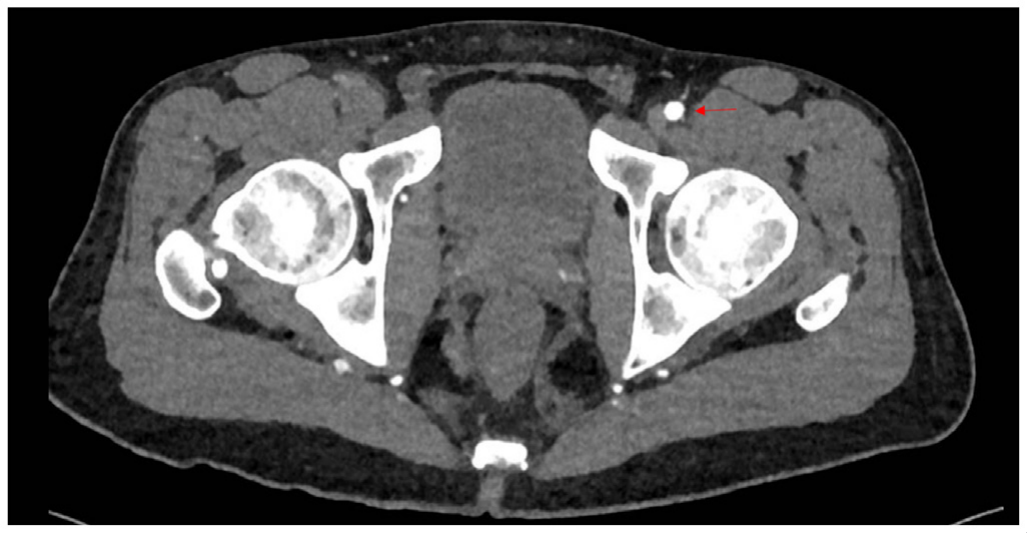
Computed tomography angiography demonstrating occlusion of the right and a normal left common femoral artery (*red arrow*).
